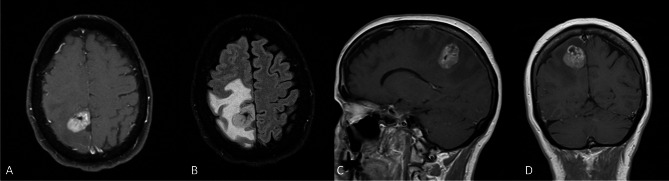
Axial T1 post-contrast image depicting a well-circumscribed, homogeneously enhancing right parietal mass in a 62 year-old woman with subjective left-sided weakness (A), axial T2 FLAIR image depicting significant perilesional edema resulting in global sulcal effacement in the ipsilateral cerebral hemisphere (B), and sagittal (C) and coronal (D) T1 post-contrast MRI also demonstrating a relatively circumscribed mass with enhancement and showing the location of the mass at the grey-white junction
